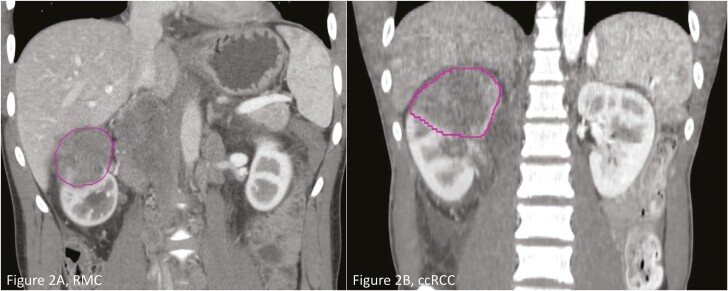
Segmented CT images. (A) RMC. (B) ccRCC.
